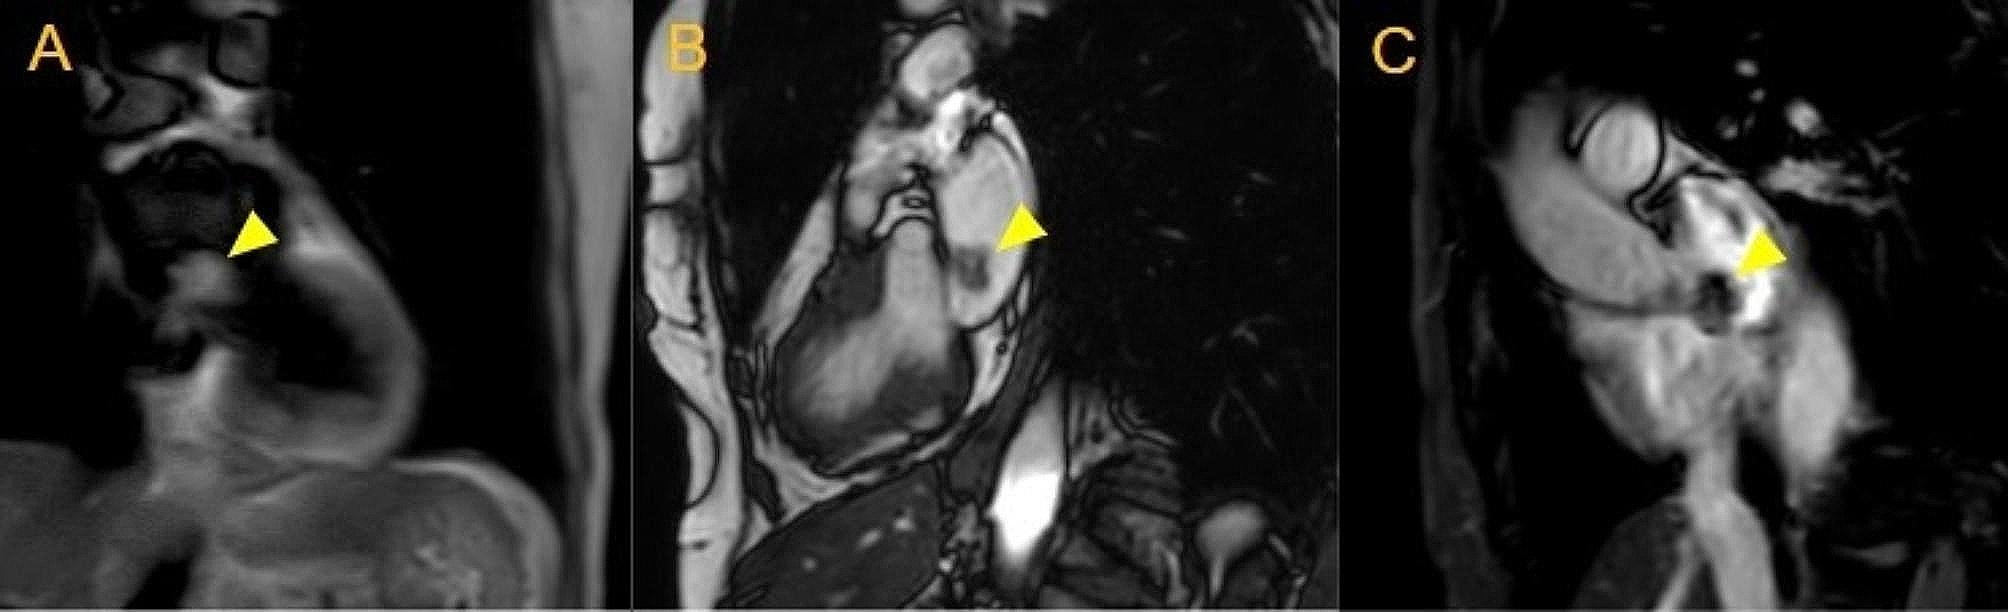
A left atrial mass (yellow arrowhead) is seen appearing isointense to myocardium on a dark blood T1-weighted spin echo coronal view (panel A), isointense/mildly hyperintense to myocardium on a balanced steady state free precession 2-chamber view (panel B), and heterogeneously hypointense on a late gadolinium-enhanced very high basal short axis view (panel C) when assessed by cardiac MRI
